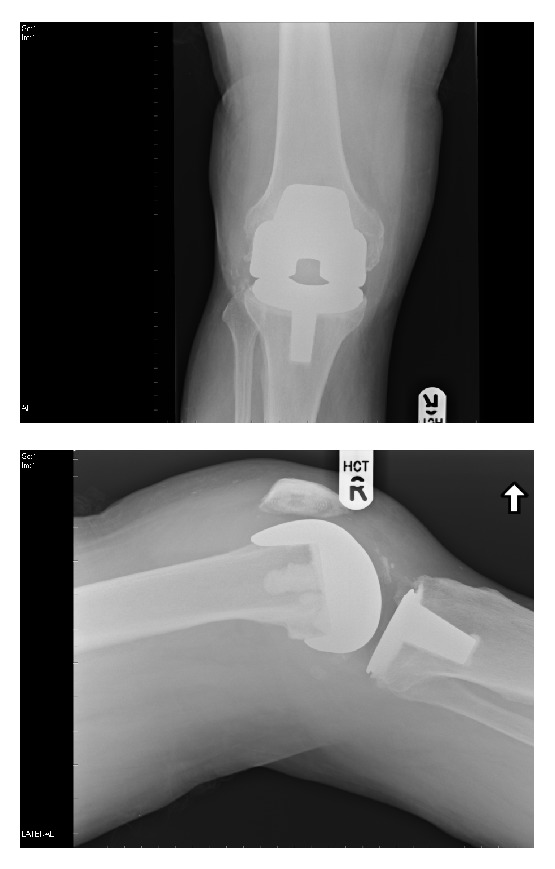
Initial operative AP and lateral radiographs of the right knee.
